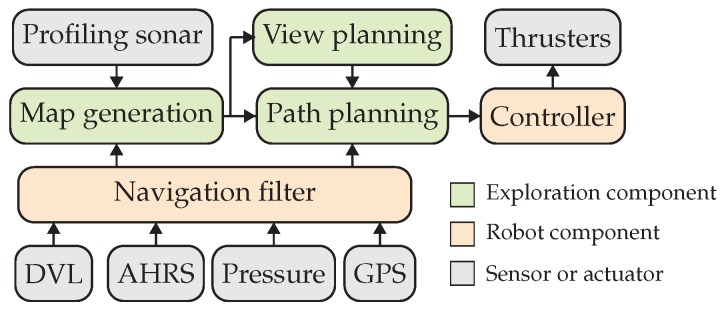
The modular design of our proposal eases integration with typical robotic software architectures. The green blocks are the components developed in our proposal. They interact with the profiling sonar sensor, the vehicle controller and the navigation block. The navigation block is in charge of the localization of the vehicle through dead reckoning, using a Doppler velocity log (DVL) sensor, an attitude and heading reference system (AHRS), a pressure sensor and a global positioning system (GPS) sensor.
